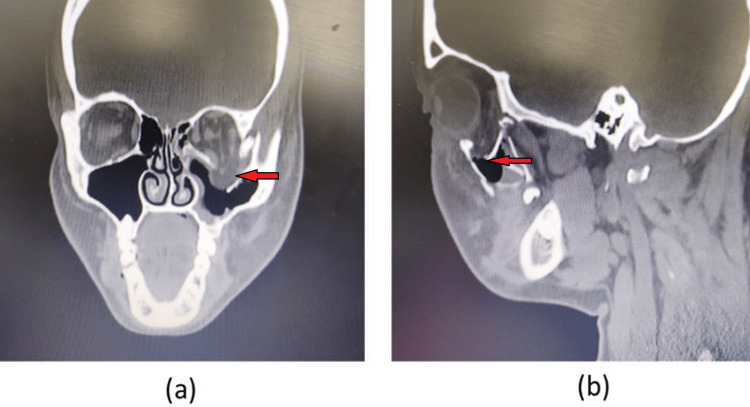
Preoperative CT images showing fracture of orbital floor (a) Coronal view; (b) sagittal view
